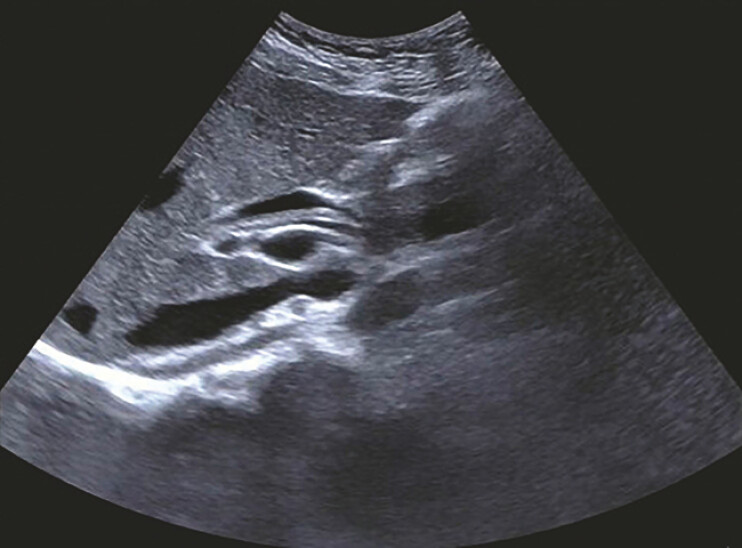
Abdominal color Doppler ultrasonography identified dual parallel linear hyperechoic structures within the biliary tract, exhibiting no acoustic shadowing.
